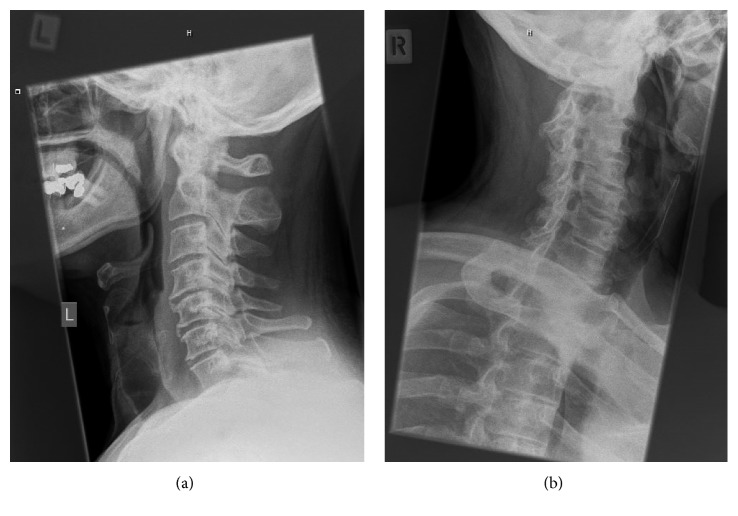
Preoperative AP and oblique cervical spine radiographs showing degenerative spondylosis.
